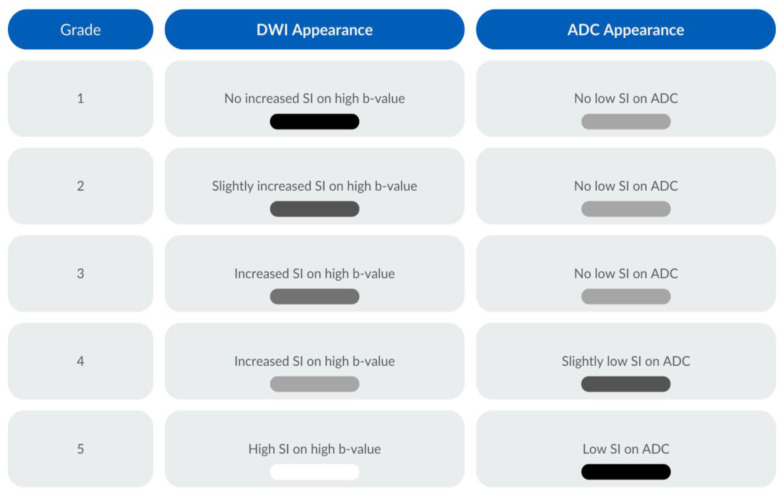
Diffusion-weighted imaging response assessment scale. The grayscale colours refer to the expected signal intensity on the DWI and the corresponding ADC map.
